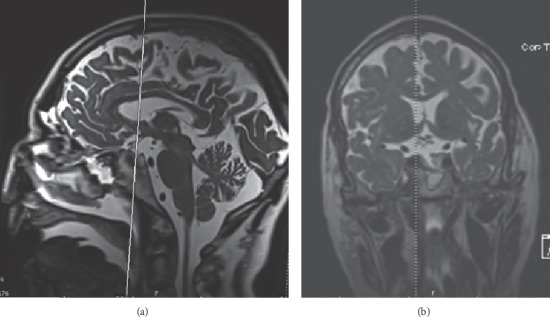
Intraoperative T2-weighted MRI: (a) sagittal and (b) coronal views following resection of paraganglioma with the cross-sectional slice indicated by the vertical lines.
